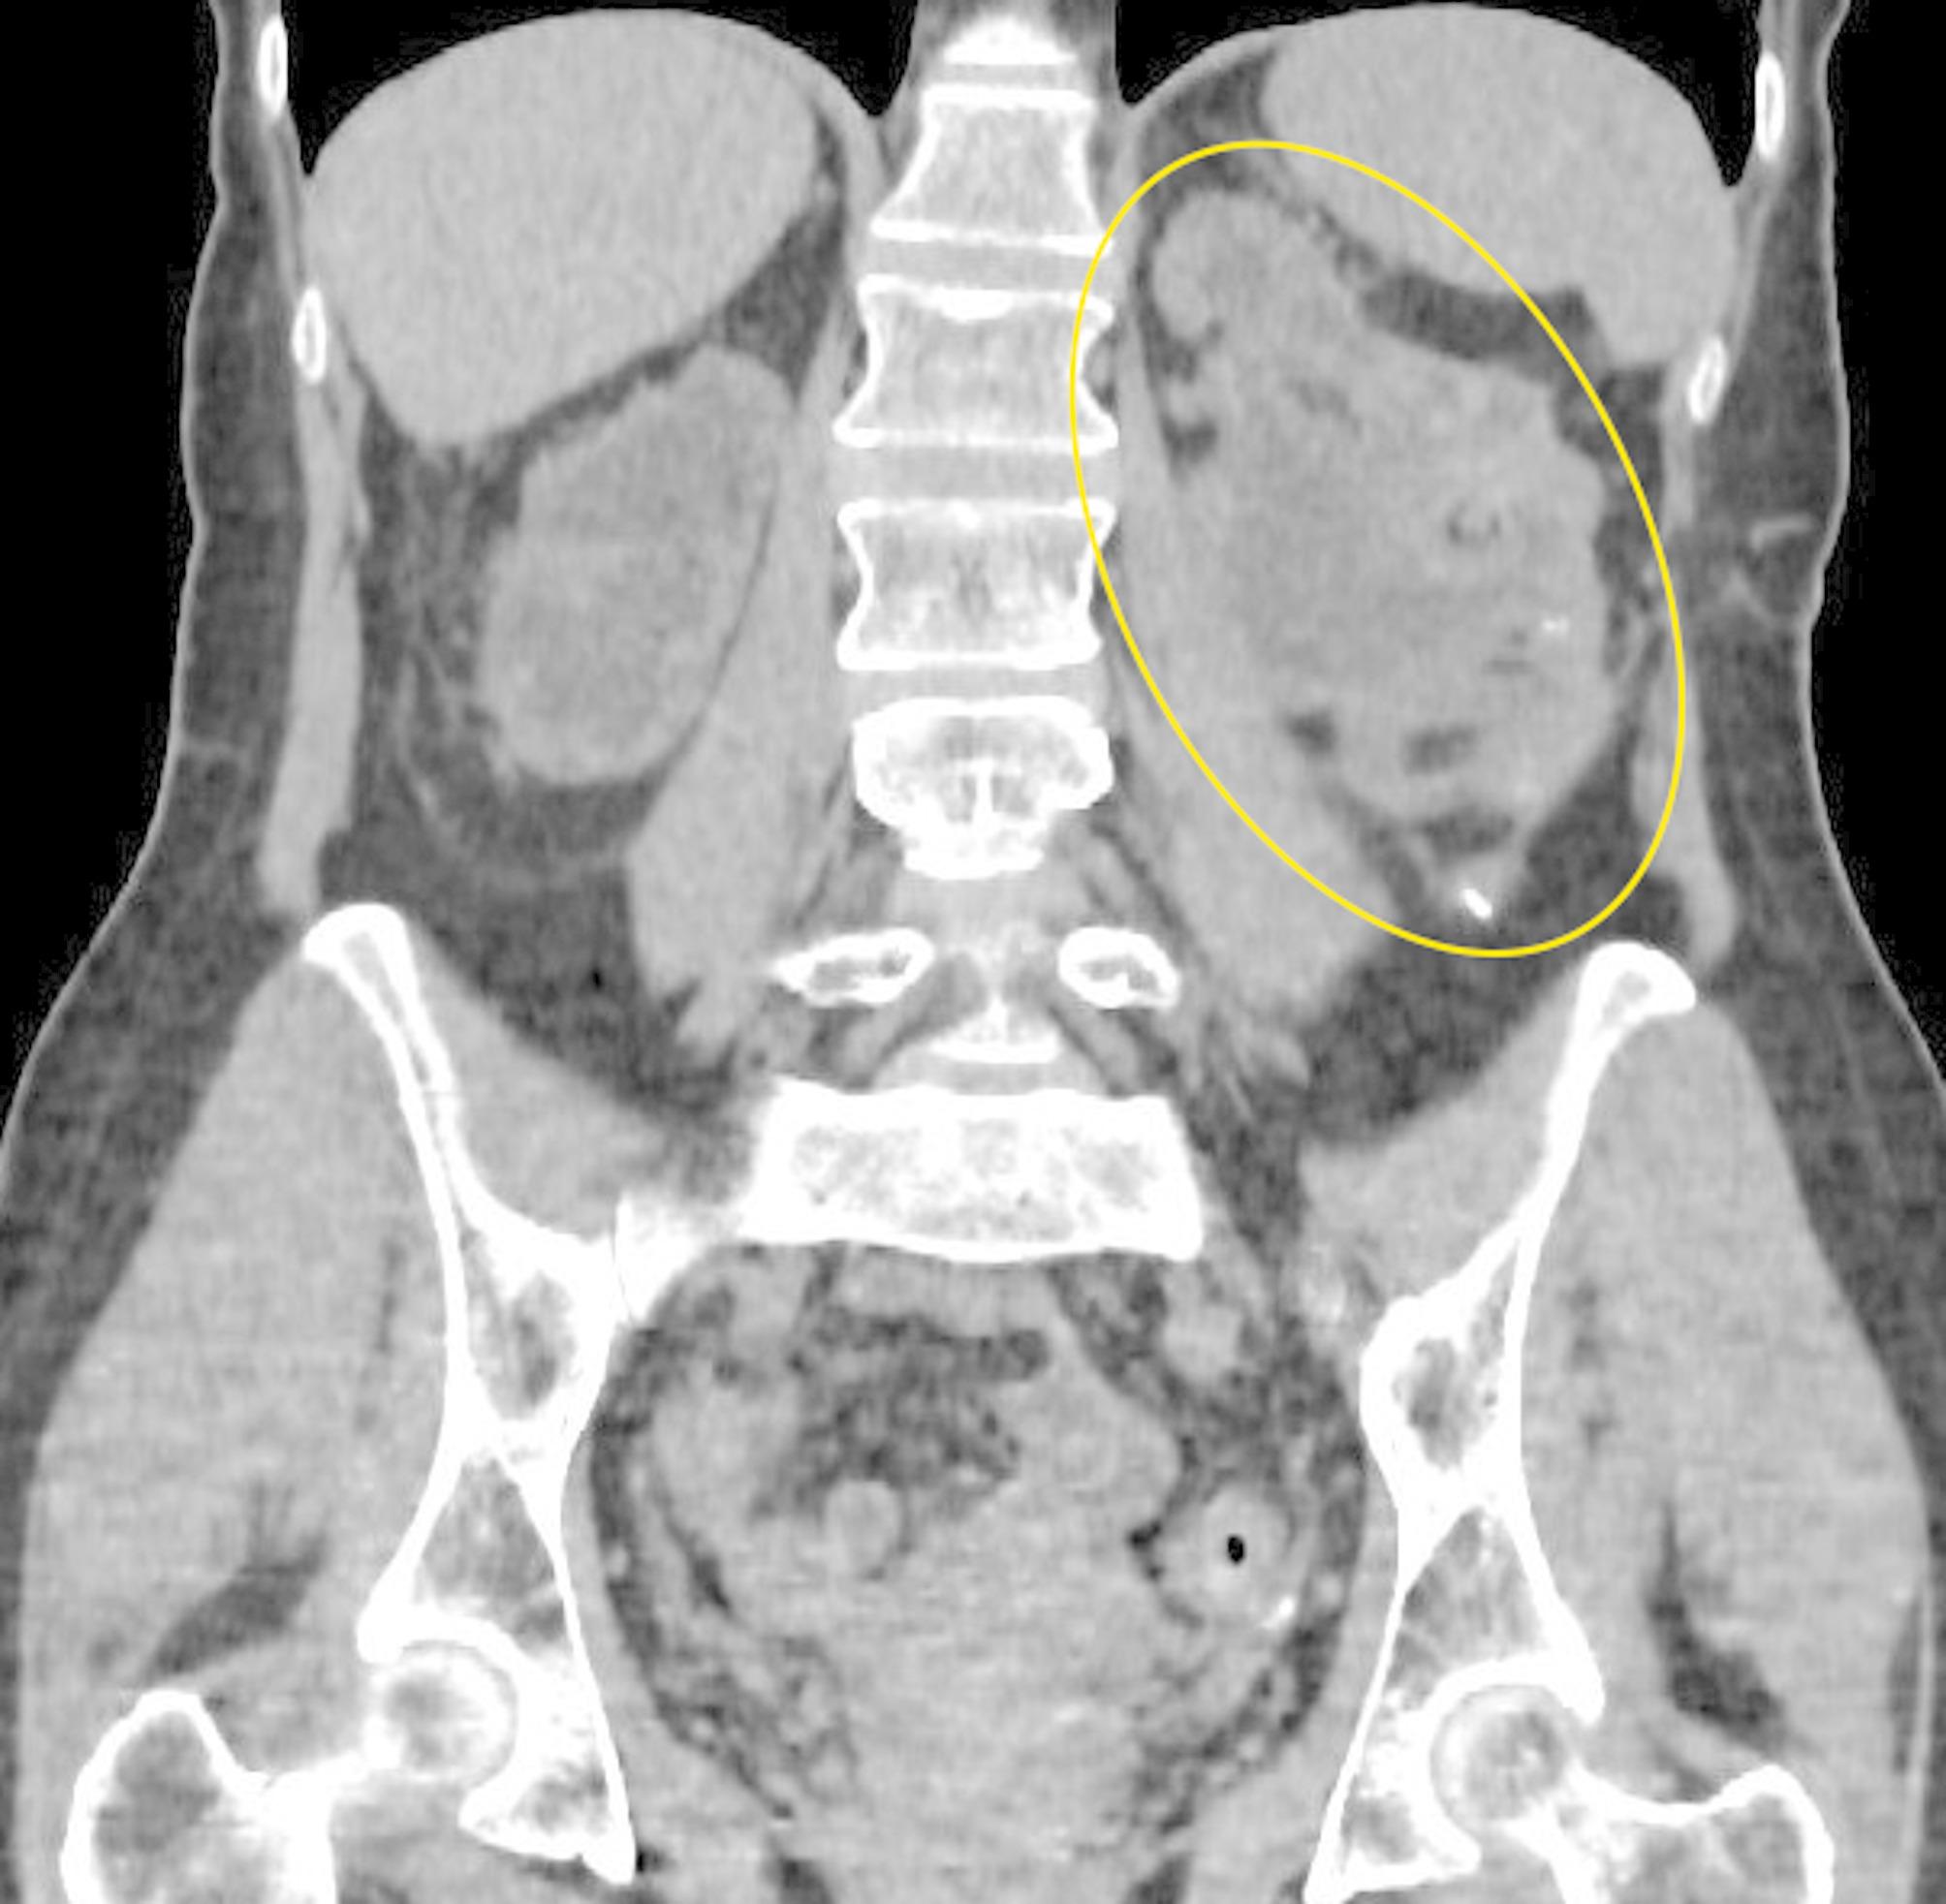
Computed tomography. The area of the left kidney is circled in yellow
